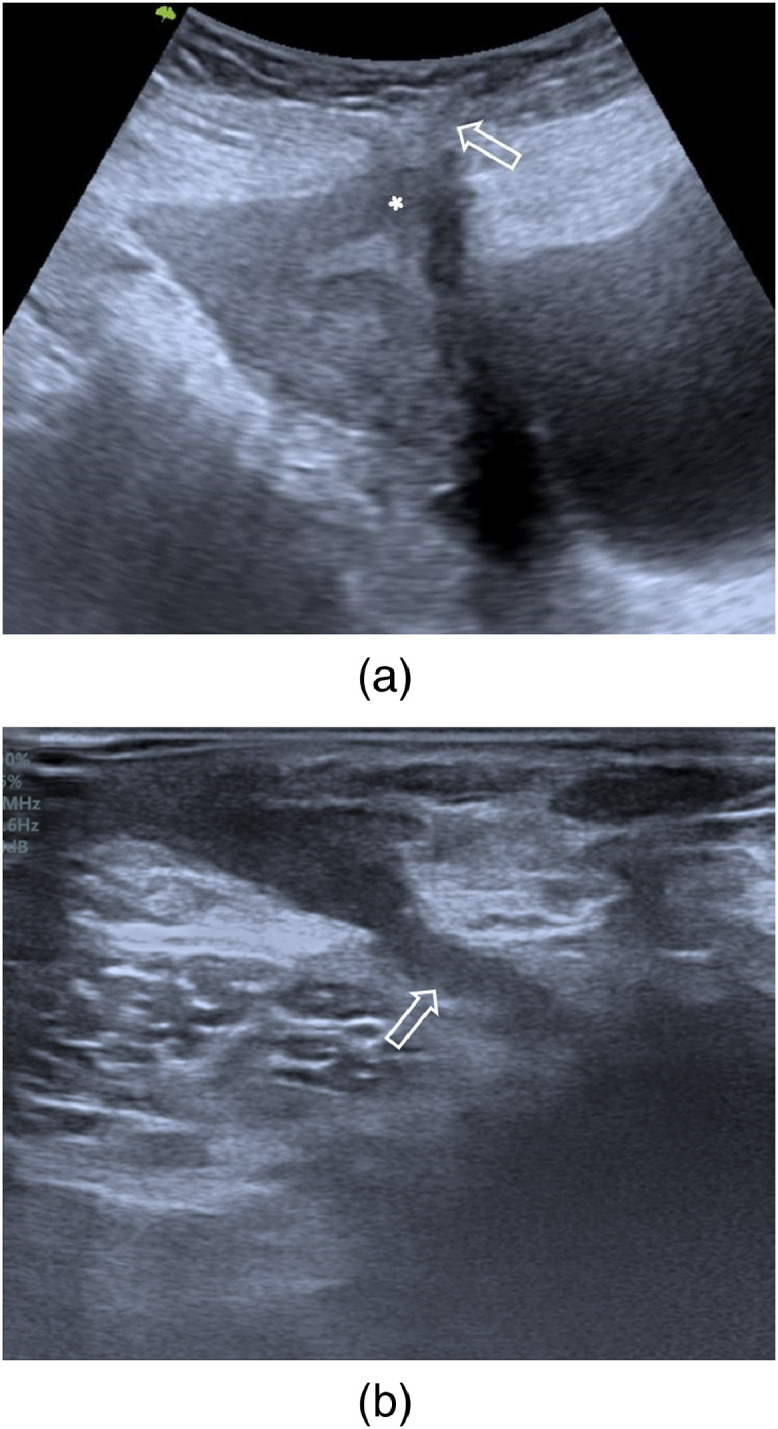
Transabdominal ultrasound with a low-frequency transducer in the sagittal plane (a) and a high-frequency transducer in the axial plane (b) showing a fistulous tract extending from the anterior body of the uterus, that shows a focal myometrial thinning related to the cesarean section scar (asterisk), to the right end of the cutaneous scar (arrow).
